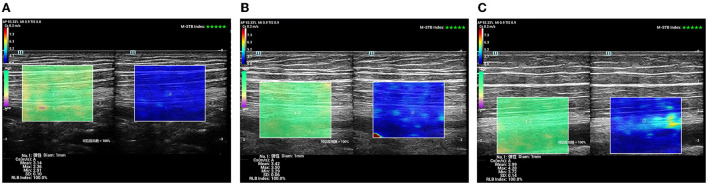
SWE sonogram of the SN. (A) Control subject; (B) patient with T2DM, non-DPN group; (C) patient with T2DM, DPN group.
